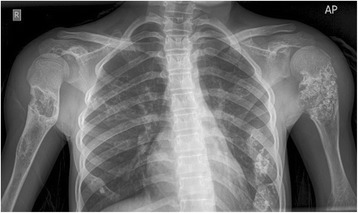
AP chest x-ray shows multiple lytic lesions with punctate calcifications in the anterior end of ribs and proximal metaphyses of both humeri extending to the diaphysis of right humerus
